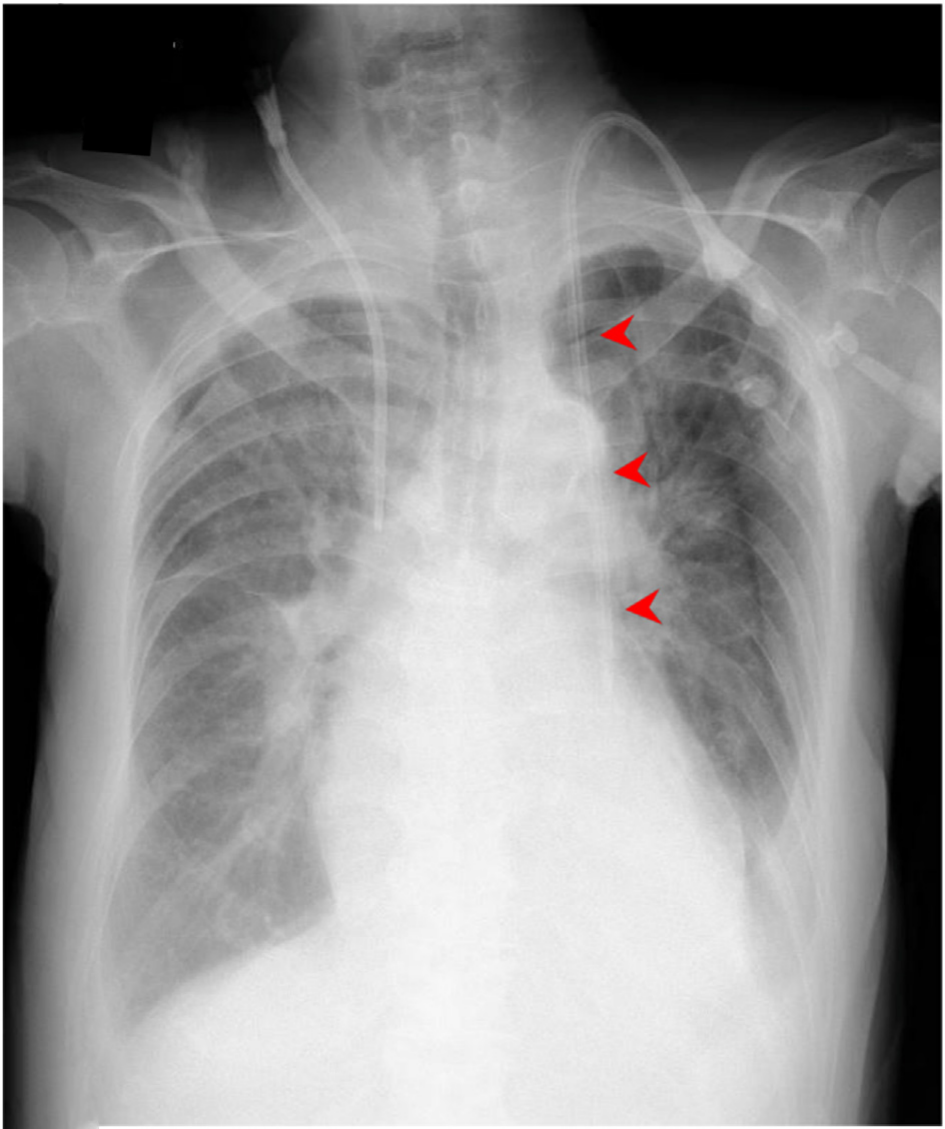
Chest radiograph showing hemodialysis catheter positioned along the left side of the mediastinum (arrow head).
